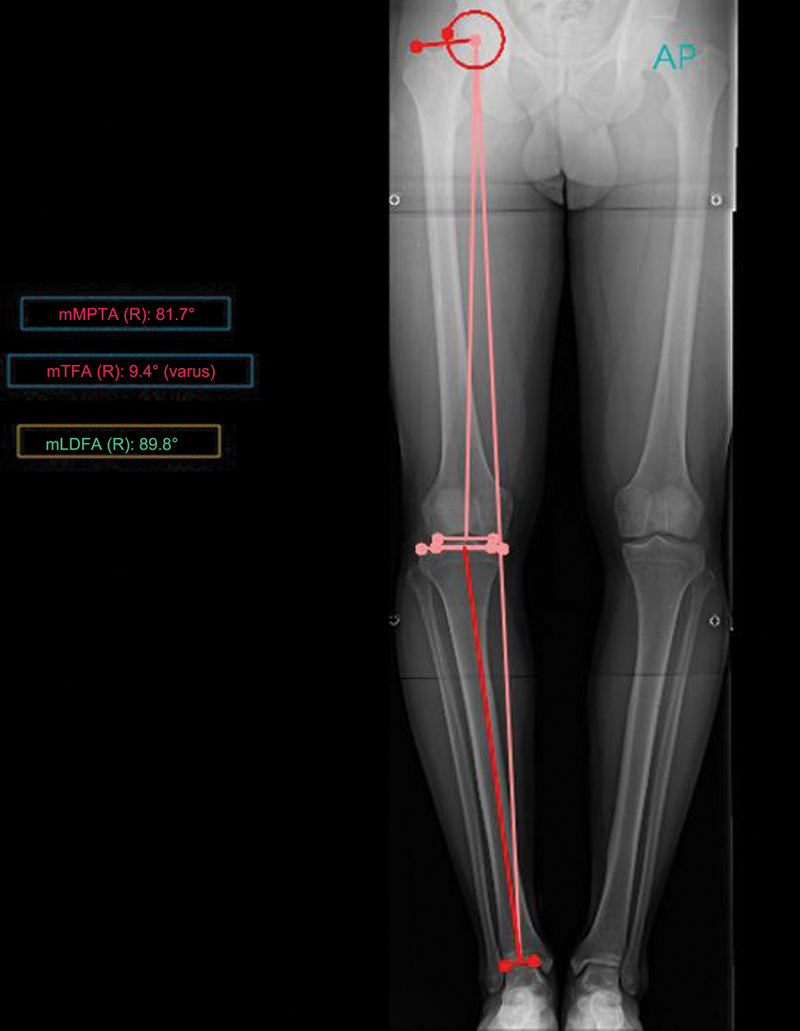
Lower limb panoramic radiograph with angular measurements.
